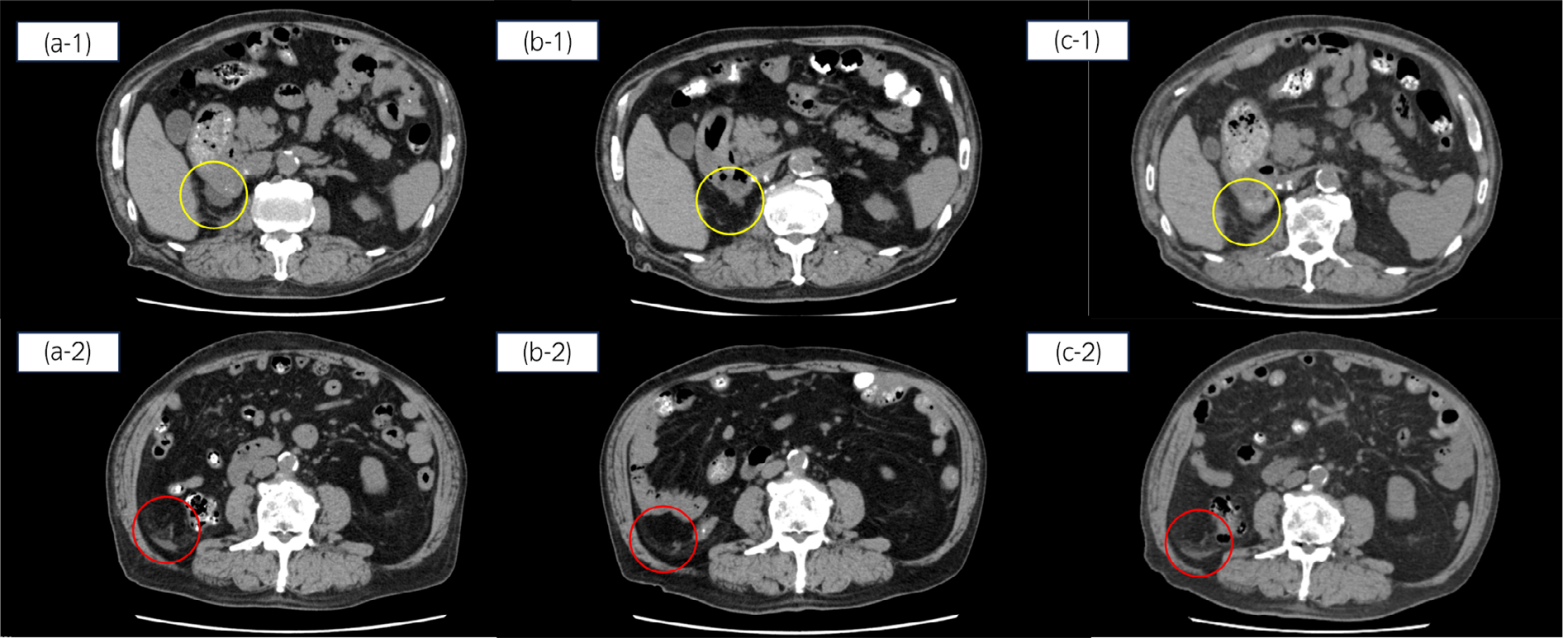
CT findings in Patient 1. CT from February X before EV treatment demonstrating disseminated two lesions of the peritoneum (yellow and red circles, a‐1, ‐2), from April X after two cycles of EV demonstrating PR of both lesions (b‐1, ‐2), and from July X after four cycles of EV demonstrating PD, which is an increase in the size of the both lesions (c‐1, ‐2).
